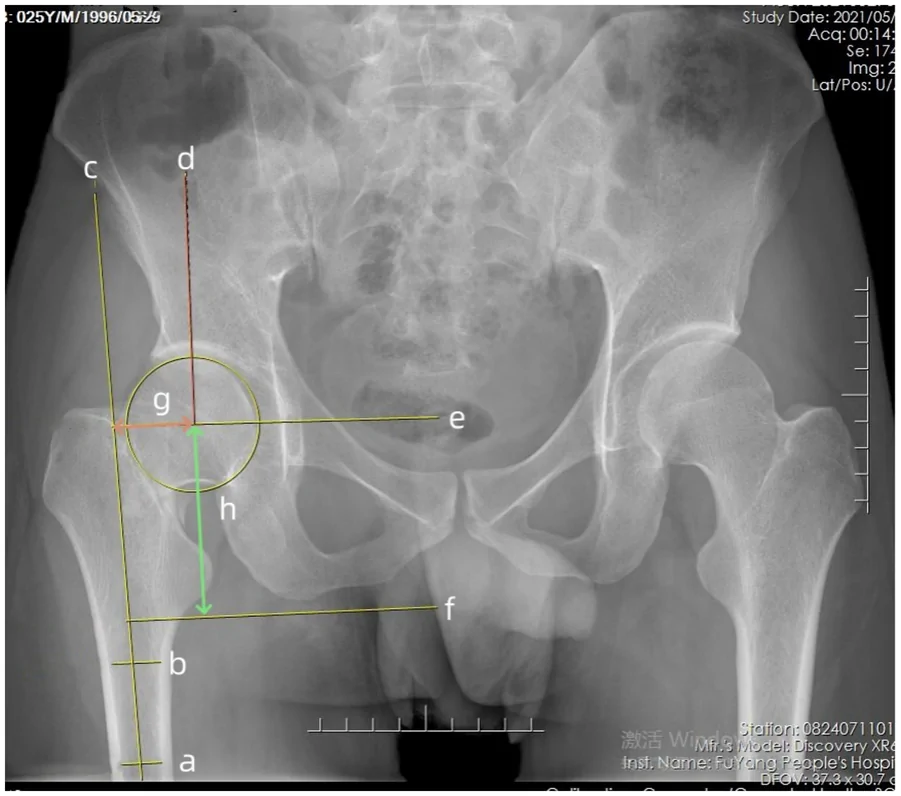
Radiographic measurement methods for femoral neck shortening and femoral offset (FO). Anteroposterior pelvic radiograph demonstrating the measurement of femoral neck shortening and FO. The axis of the femoral shaft (c) was defined by the midpoint of the femoral medullary cavity (a,b). The perpendicular distance from the femoral head center (d) to the femoral shaft axis (c) represented femoral offset (FO). The distance (h) between the center of the femoral head (e) and the upper edge of the lesser trochanter was measured perpendicular to the femoral shaft axis (c) to evaluate femoral neck length. FO, femoral offset.
